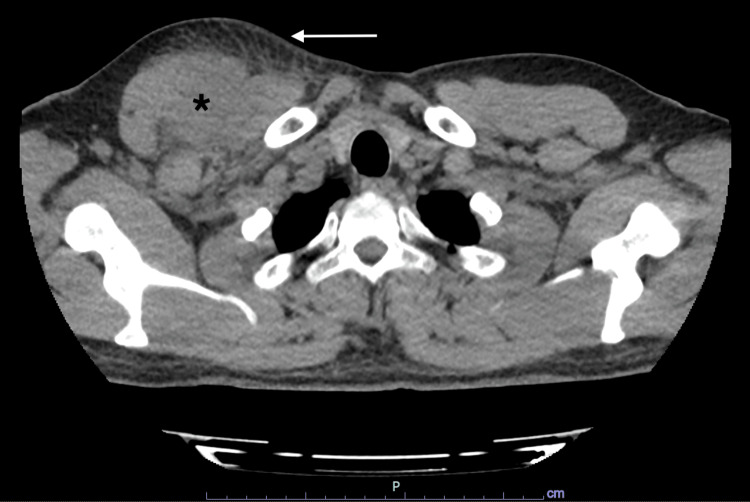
Thoracic CT scan exhibiting asymmetric and heterogeneous hypertrophy in the right pectoral muscle (asterisk) and adjacent subcutaneous tissue densification (arrow)
